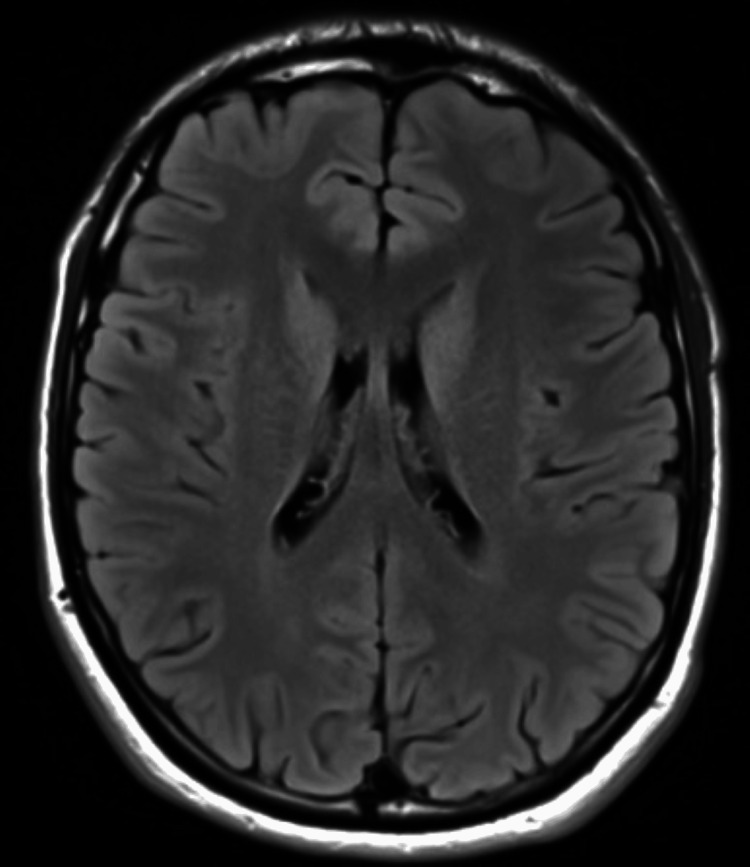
Normal brain MRI demonstrating no abnormalities.
